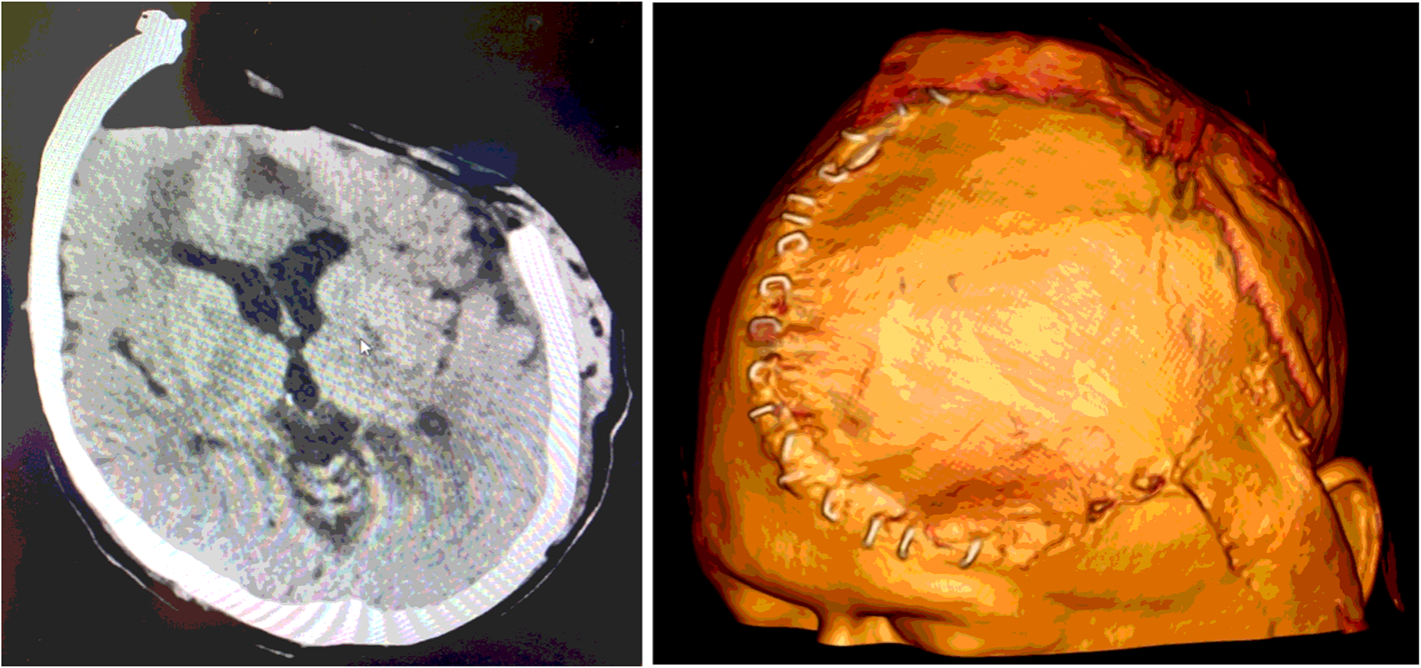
CT head scan with 3D reconstruction before device application
